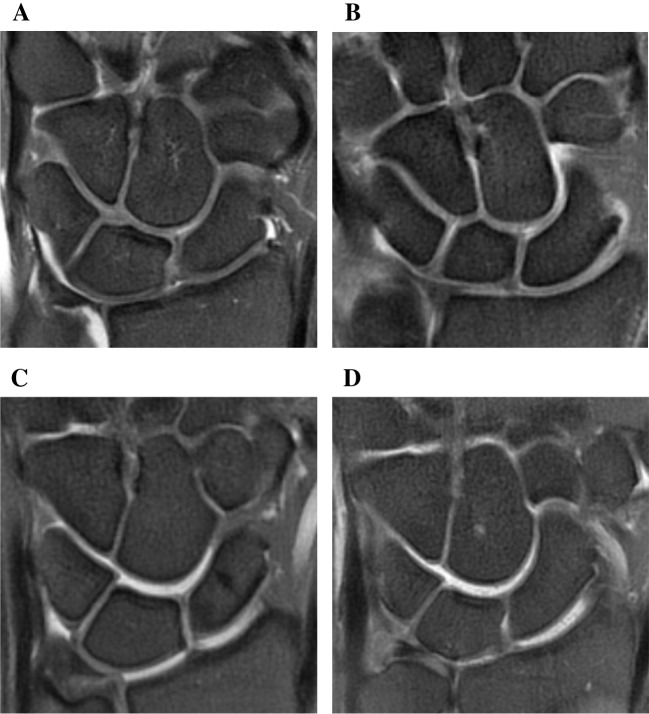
PDFS coronal left wrist MR images: (A) 27- and (B) 44-year-old female wrists without traction, (C) 28-year-old female and (D) 41-year-old male wrists with traction. Joint space width was increased in the radioscaphoid, radiolunate, scaphocapitate, lunocapitate, and triquetrohamate joints after traction
